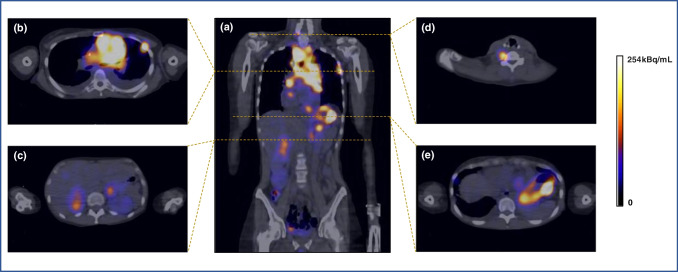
[^177^Lu]Lu-DOTA-MGS5 SPECT/CT scan at 24 h p.i. (a) fused coronal view of the full body scan. Dashed areas indicate the level of the axial slices at (b) left-central primary tumour and cervical/mediastinal lesions, as well as a left subpleural lesion, (c) both adrenal glands lesions, (d) right cervical vertebral metastasis and (e) soft tissue lesion in the gastric curvature together with physiological uptake in the gastric wall. The scale is set at 30% of the maximum activity concentration of 845 kBq/ml
